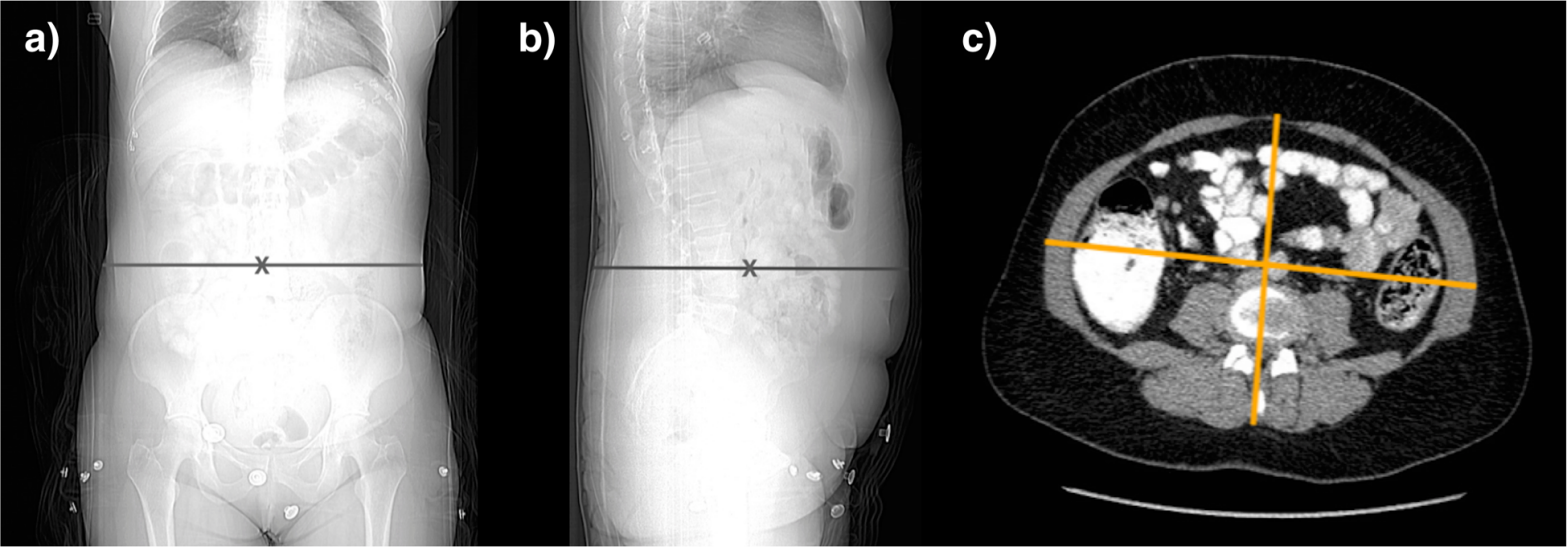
Measurement of lateral (*D*_*LAT*_) (a) anteroposterior (*D*_*AP*_) (b) skin-to-skin patient diameters at the midslice level on CT-localiser images. Measurement of the inner lateral and anteroposterior diameters on the axial midslice CT image excluding the subcutaneous adipose tissue (c) to allow calculation of the effect inner diameter (*D*_*IN*_) and the effective diameter ratio (*D*_*RATIO*_)
